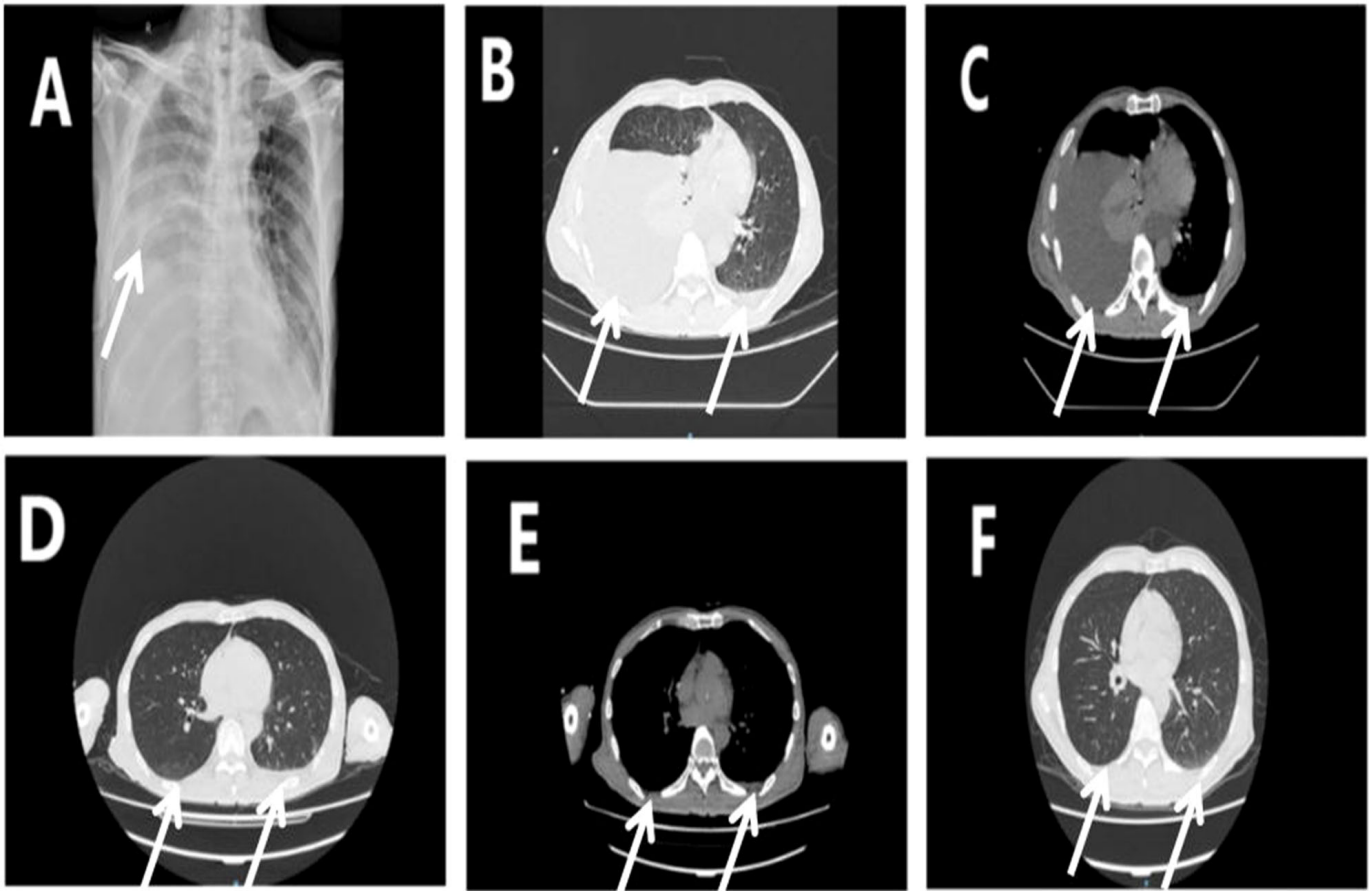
Radiologic changes of the chest before and after treatment. (A) Chest X-ray on POD 5 showing massive right pleural effusion (white arrow). (B, C) Chest CT on POD 14: lung window (B) and mediastinal window (C) showing massive right and mild left pleural effusions (white arrows). (D, E) Chest CT on POD 35: lung window (D) and mediastinal window (E) showing significant reduction in right-sided effusion (white arrows). (F) Chest CT at 3-month follow-up (lung window) showing near-complete resolution of bilateral pleural effusions (white arrows).
